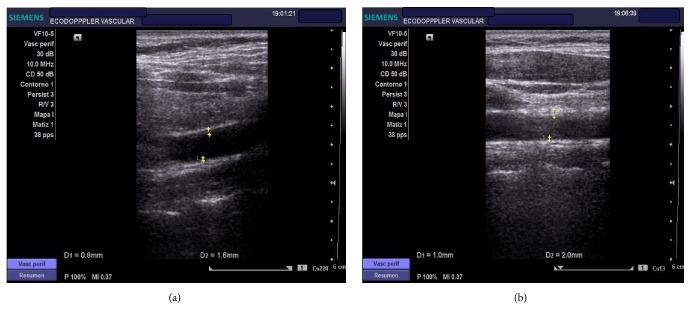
The vascular ultrasound study in our patient showed atherogenic plates: (a) 1.6 mm in RCCA (right common carotid artery) and (b) 2 mm in LCCA (left common carotid artery).
